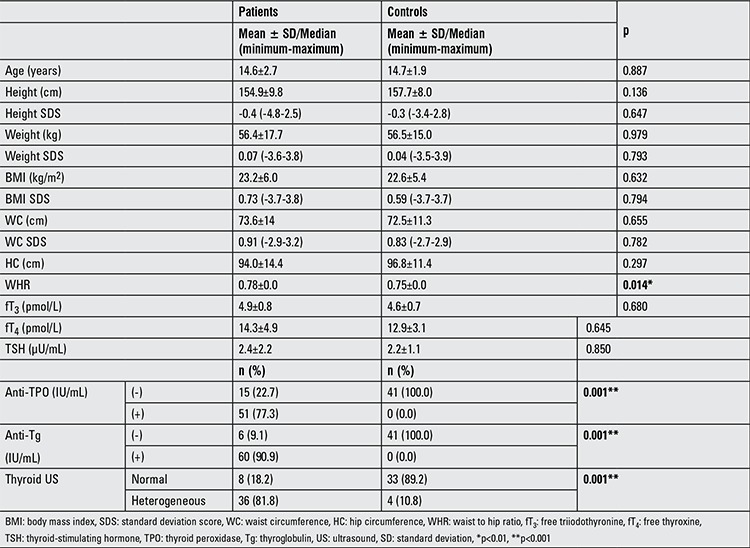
Anthropometric characteristics, thyroid antibody levels, and results of thyroid function tests and thyroid ultrasonography in the patients and in controls
